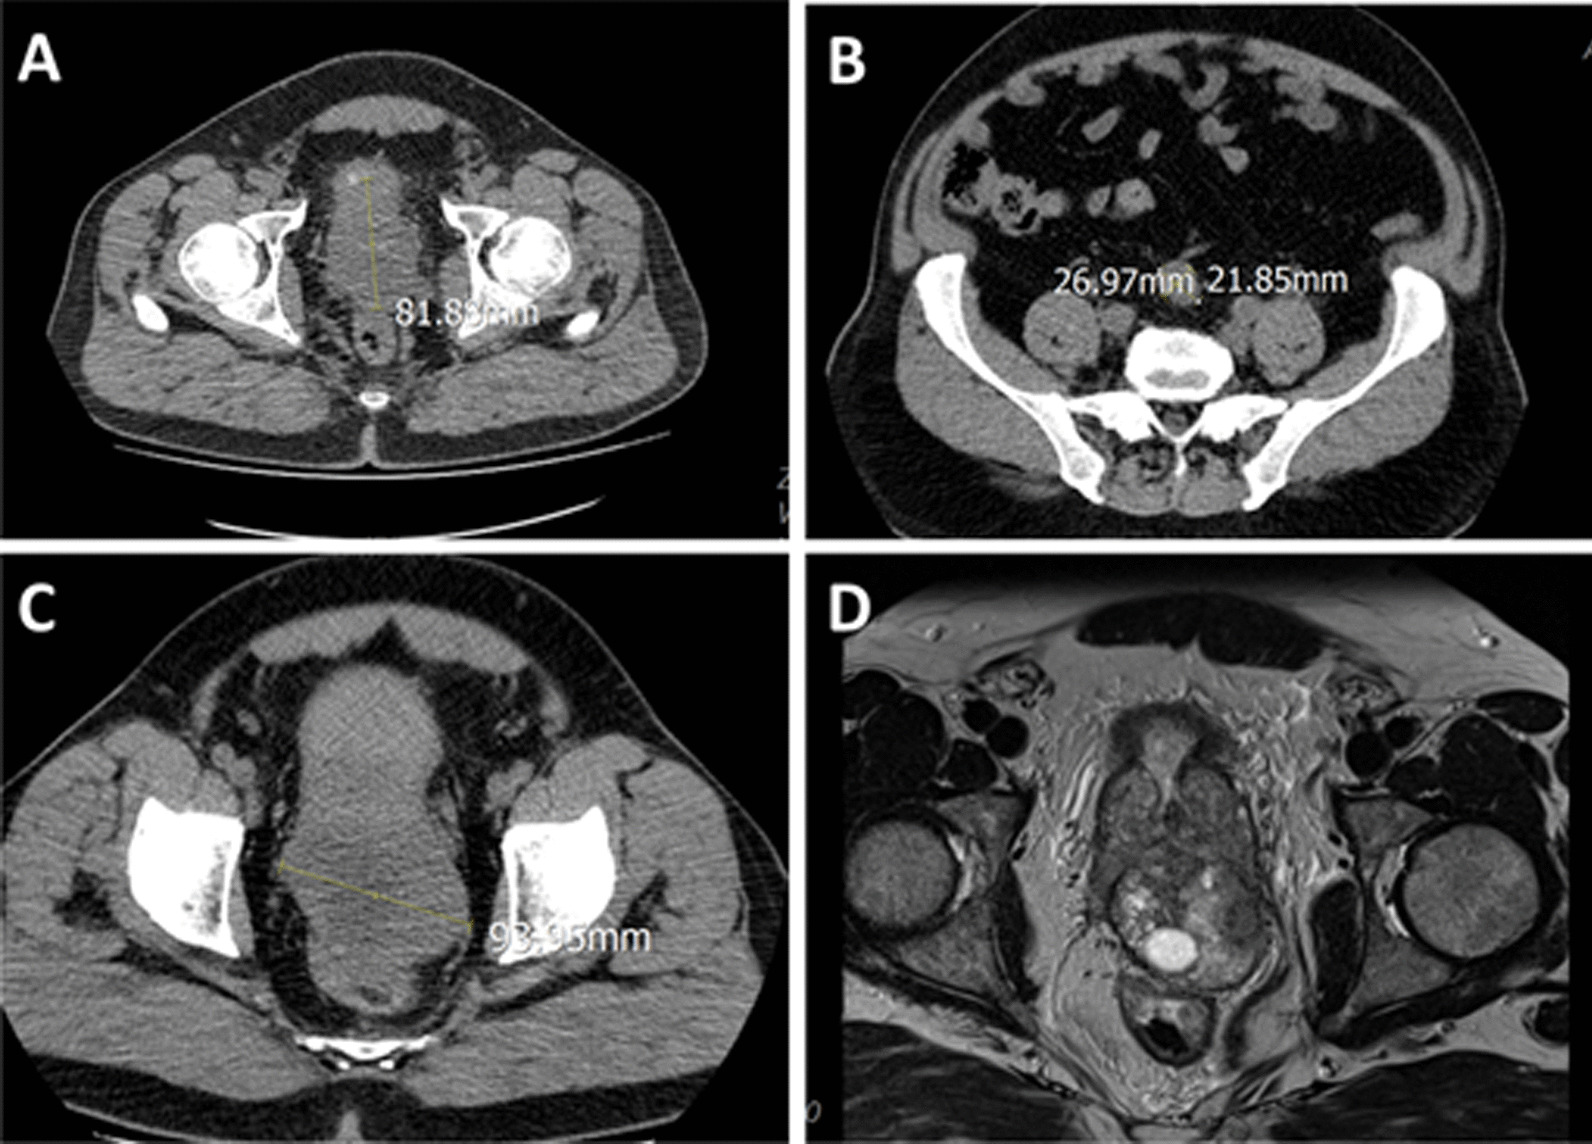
Imaging studies of patient’s urothelial tumor after progression on computed tomography (CT) (A–C) and magnetic resonance imaging (MRI) (D). A Longitudinal dimension of the tumor. B Short-axis diameter of pelvic lymph node. C Transverse dimension of tumor. D MRI of patient’s tumor in pelvis
